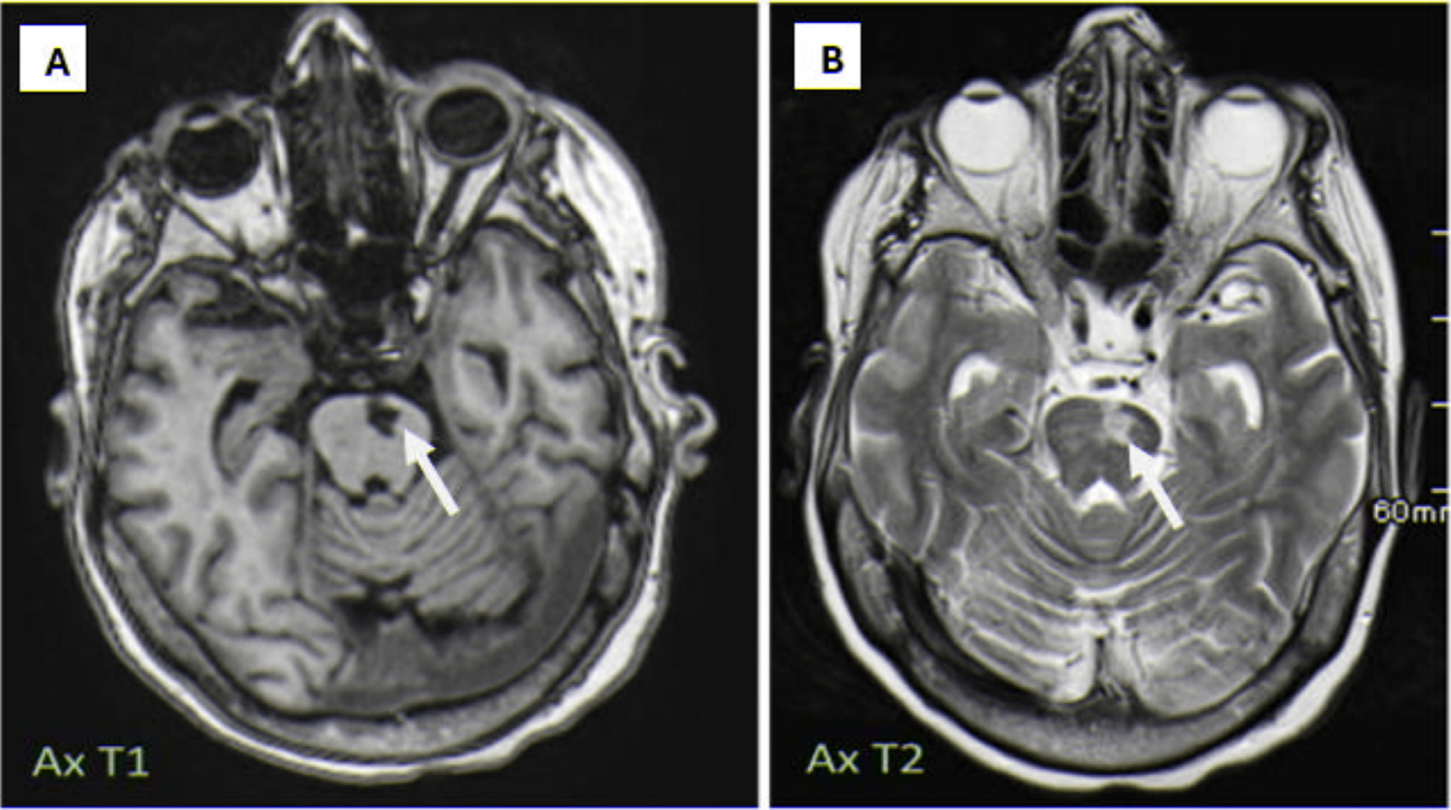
Brain MRI demonstrating chronic left paramedian pontine infarction. (A) Axial T1-weighted image showing a hypointense signal in the left paramedian pons (white arrow). (B) Axial T2-weighted image revealing a hyperintense signal in the corresponding region (white arrow), confirming the chronic nature of the infarct.
